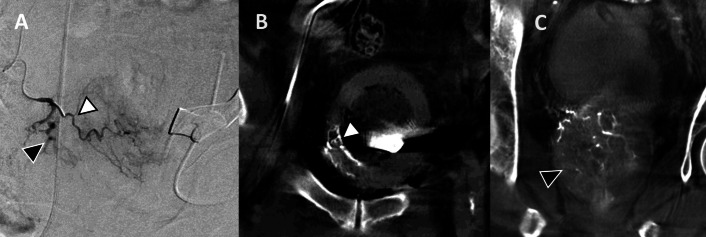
A Initial DSA of right internal iliac artery demonstrated a typical prostatic artery origin from the superior vesical artery and a possible prostate blush (open arrowhead). B However, CBCT demonstrates that this blush was the thickened bladder wall (white arrowhead). C The more proximal branch (black arrowhead in panel A) of this same artery was in fact perfusing the prostate (black arrowhead)
